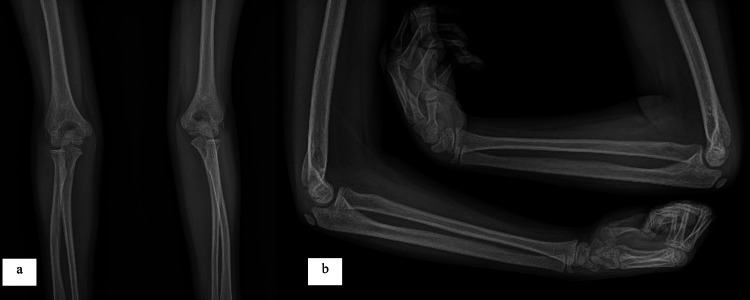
a) Last follow-up examination (second year) comparative elbow AP X-ray. b) Last follow-up examination (second year) comparative elbow lateral X-ray
